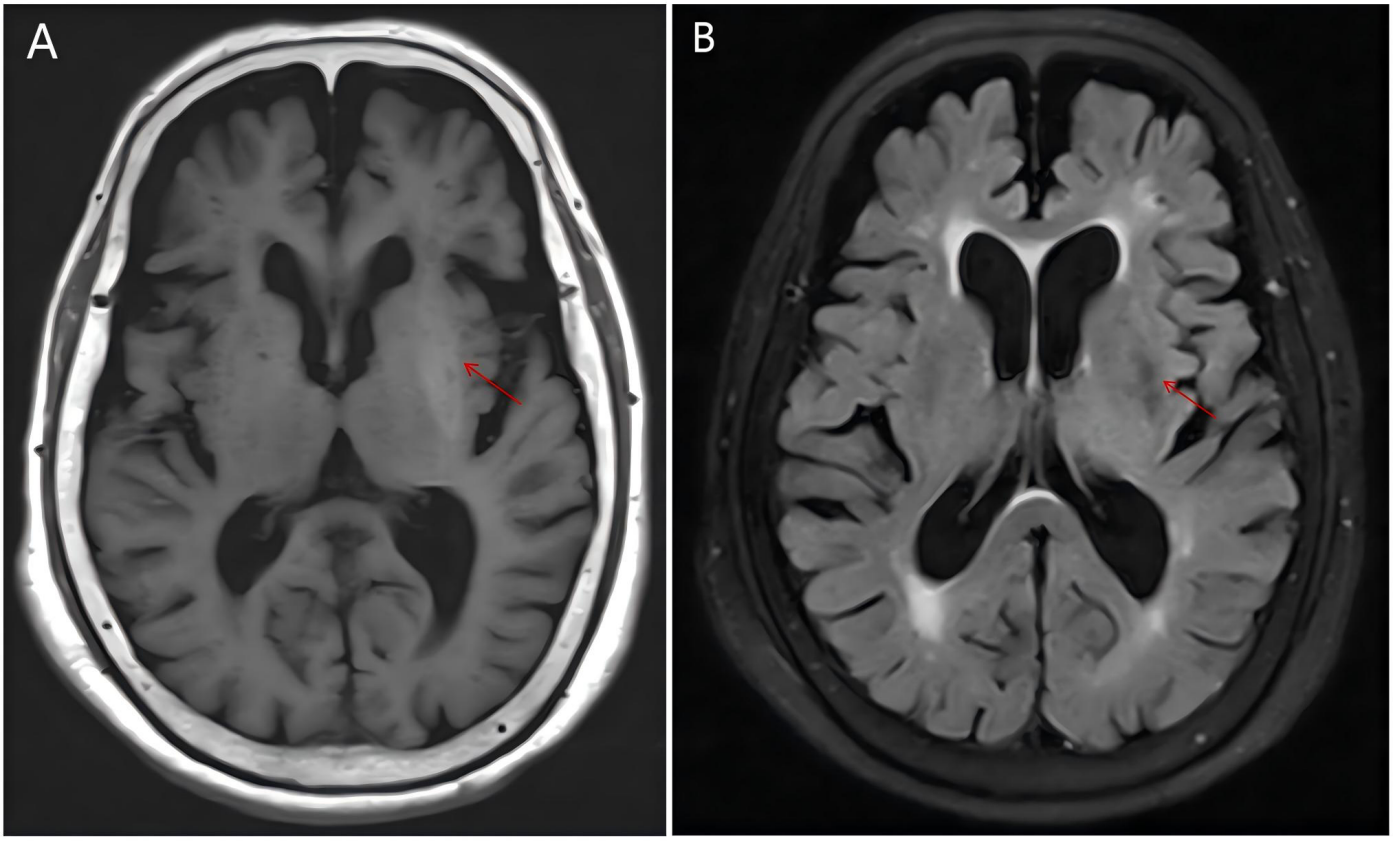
The MRI scan reveals patchy short T1 (A) and slightly short T2 signal shadows in the left basal ganglia (B), with slightly high signal on the water suppression sequence, and no diffusion restriction. Abnormal signals are marked with red arrows.
